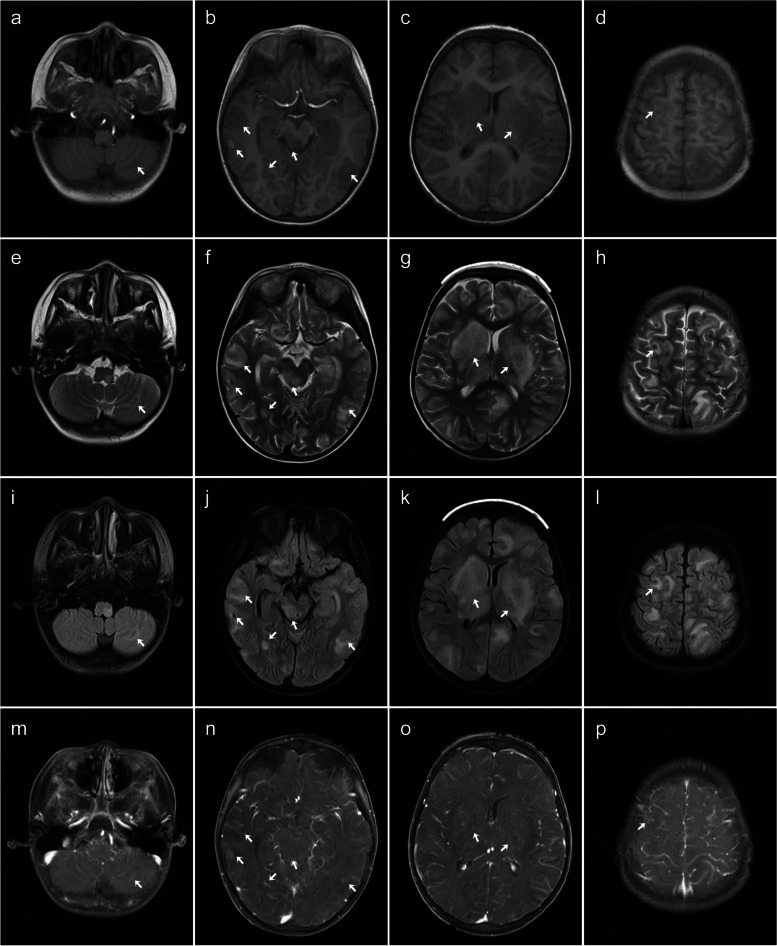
Brain MRI findings for case 4. Brain MRI with axial T1-weighted imaging (a–d), T2W-weighted imaging (e–h), and T2-fluid-attenuated inversion recovery image (i–l) showing multiple intraparenchymal lesions (white arrows) and the T2-weighted image and T2-fluid-attenuated inversion recovery images (e–l) showing extensive peri-lesional edema (white arrows). The enhance scan (m–p) showing mild to moderate heterogenous enhancement of the lesions (white arrows)
